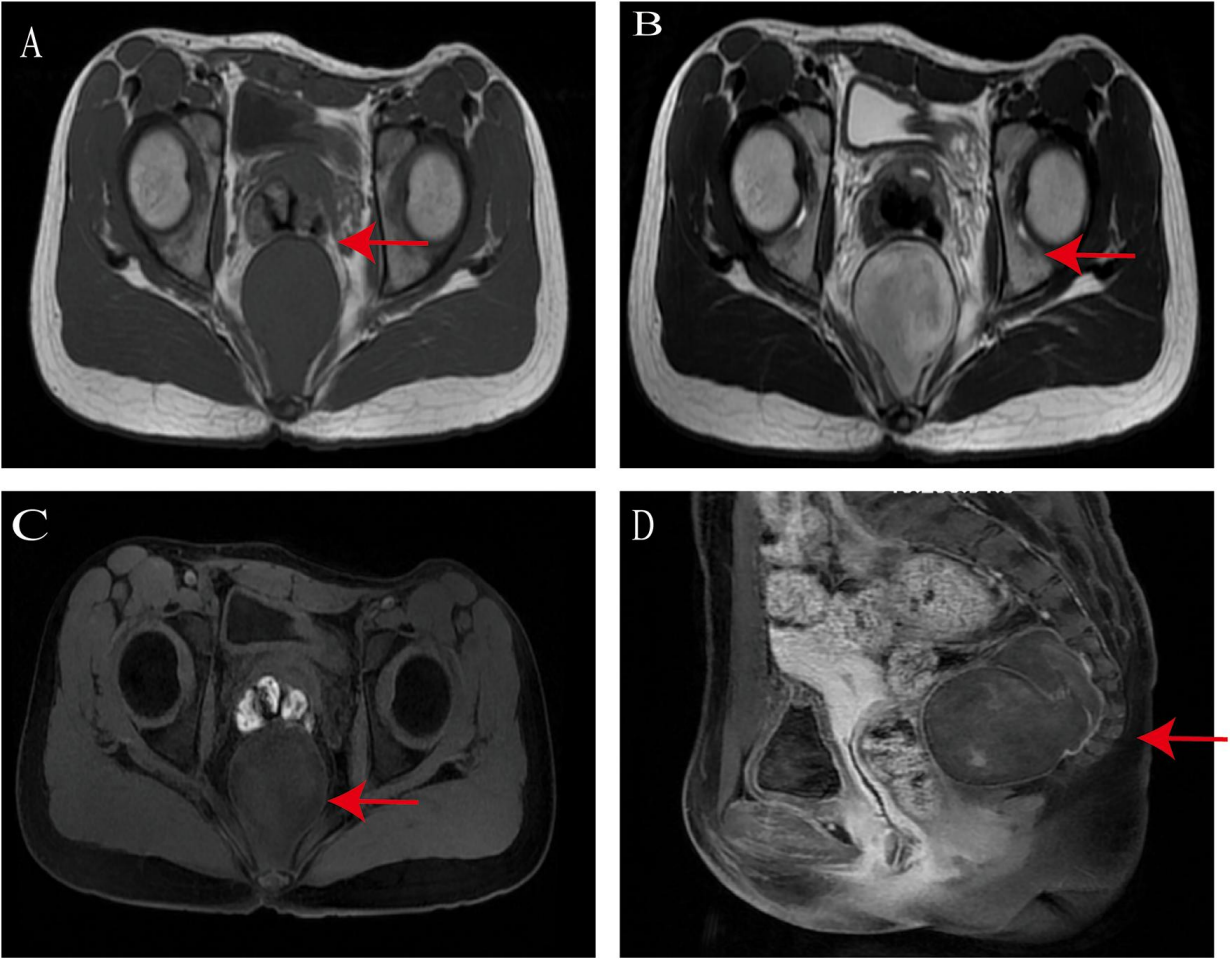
Preoperative MRI findings (May 22, 2022) (A) T1: Low signal intensity; (B) T2: high signal intensity with heterogeneous intensity; (C) T1 FSE; (D) contrast-enhanced scan shows mild heterogeneous enhancement compared to T1 FSE.
